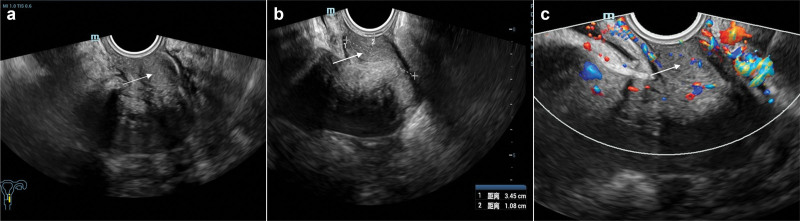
TVUS images. (A) A mass on the left vaginal wall (white arrow); (B) the mass measures 3.5 × 1.1 cm, with clear boundaries and a relatively regular shape; (C) color Doppler shows blood flow signals within the mass. TVUS = transvaginal ultrasound.
